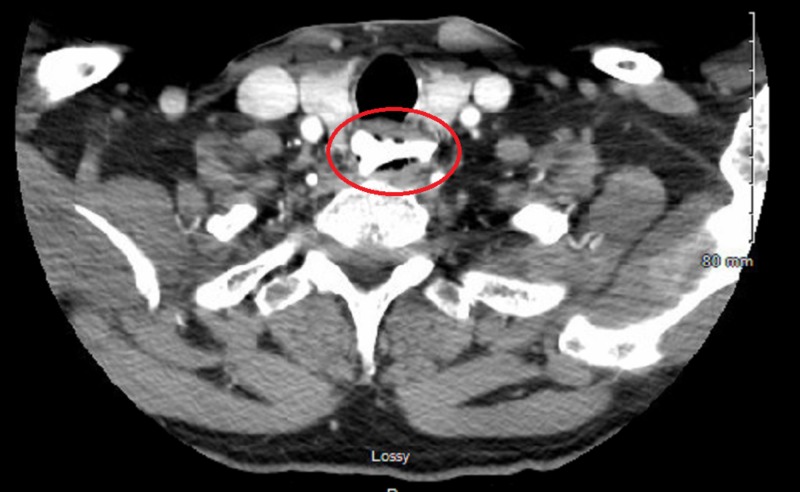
Computed tomography (CT) of neck showing a bone in the esophagus at the level of cervical vertebra 7-thoracic vertebra 1
